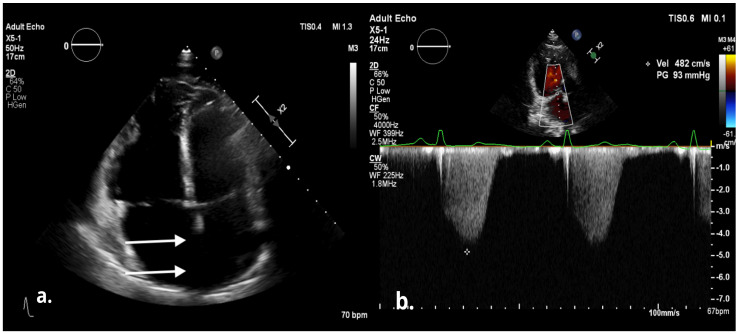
Transthoracic echocardiography imaging shows the dilatation of right heart chambers, atrial septal defect (marked by the arrows) (a) and tricuspid regurgitation with high maximal regurgitant velocity (b).
